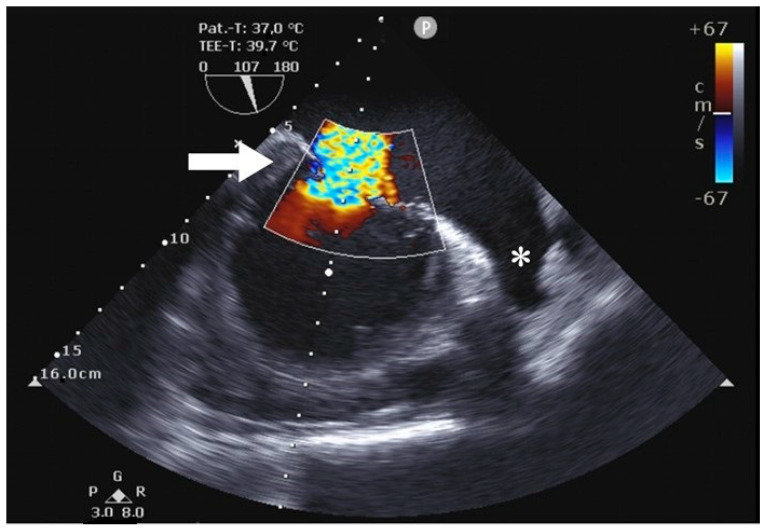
Preoperative transesophageal echocardiography showing severe mitral valve regurgitation (arrow) as well as the LAA (*).
